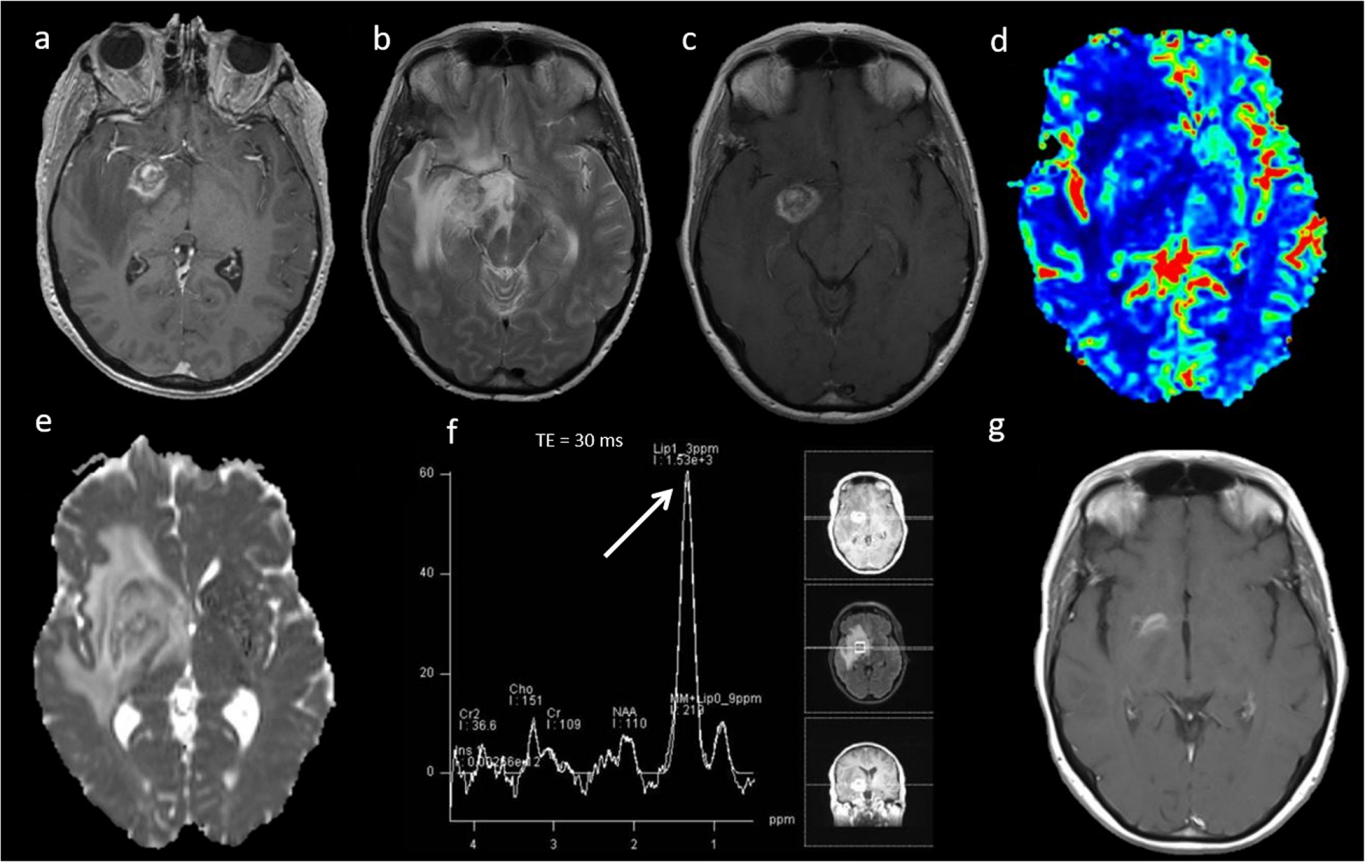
Brain metastasis—treatment effect. Melanoma metastasis. a Enhancing lesion in the right basal ganglion on axial T1 post-contrast MRI. b, c Post-SRS T2W and post-contrast T1W images shows increase in lesion size and oedema. Multiparametric MRI demonstrates: (d) an intermediate rCBV ratio (< 2.1) on PWI, (e) a high ADC (1159 × 10^−6^ mm^2^ s^−1^) and (f) low Cho/Cr ratio (1.6) and very high lipids (arrow) suggesting necrosis. g Three-month follow-up post-contrast T1W scan showed regression of the lesion
